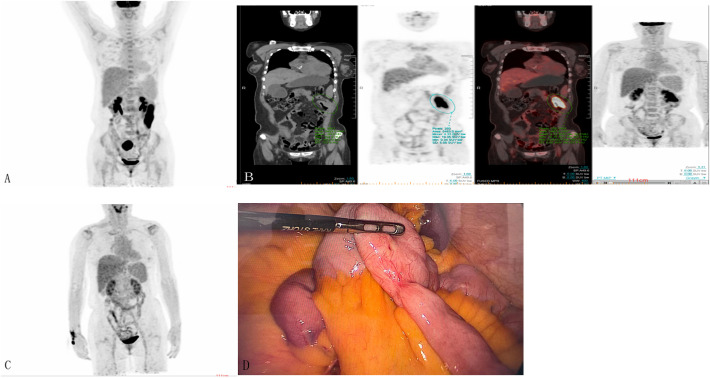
(A) The PET-CT scan at diagnosis (SUVmax 16.2). (B) The PET-CT scan at disease progression (SUVmax 20.33). (C) The last PET-CT scan for response evaluation. (D) A stenotic segment of the small intestine was confirmed by laparoscopic examination.
